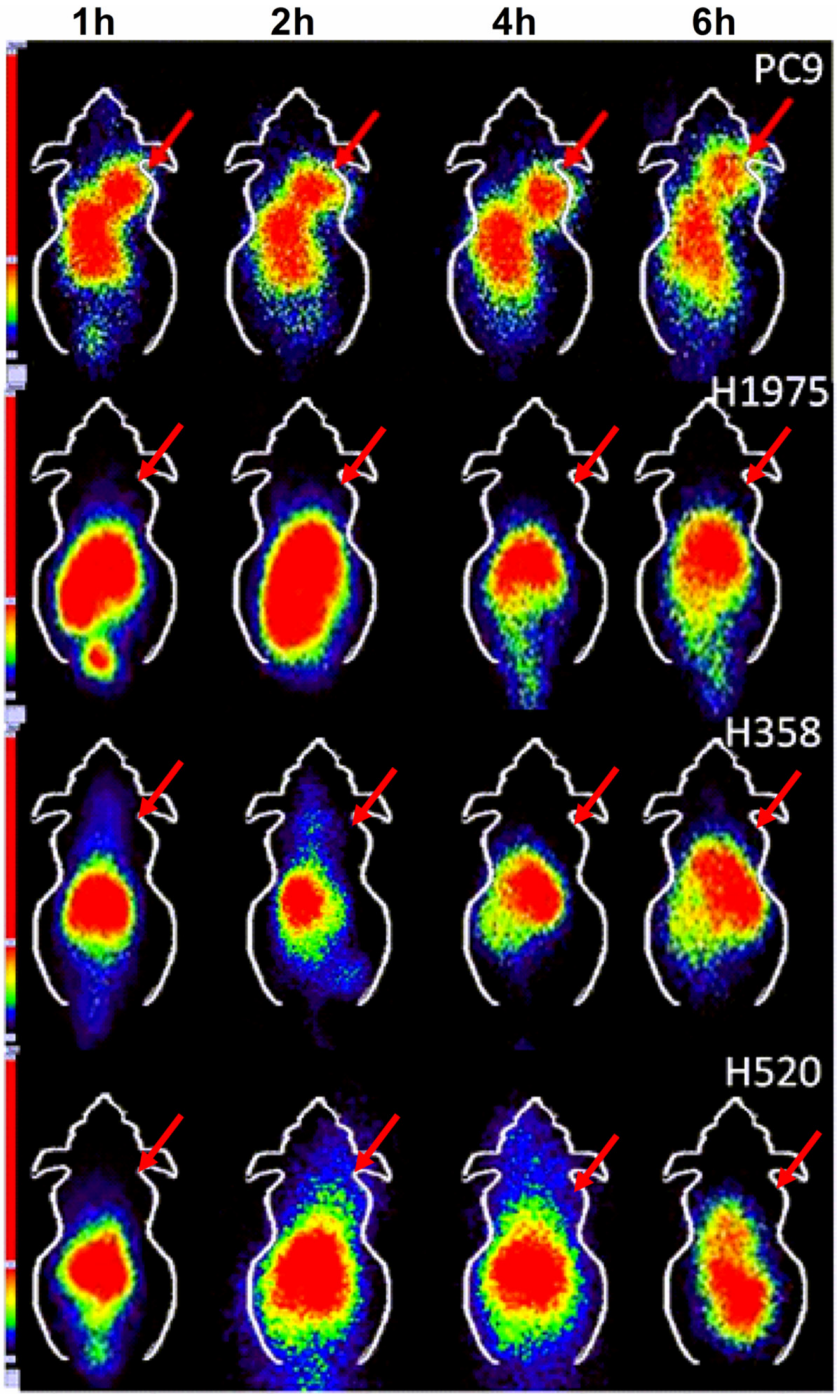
SPECT imaging of ^99m^Tc-HYNIC-MPG in PC9, H1975, H358 and H520 xenografts at 1 h, 2 h, 4 h, and 6 h time points
